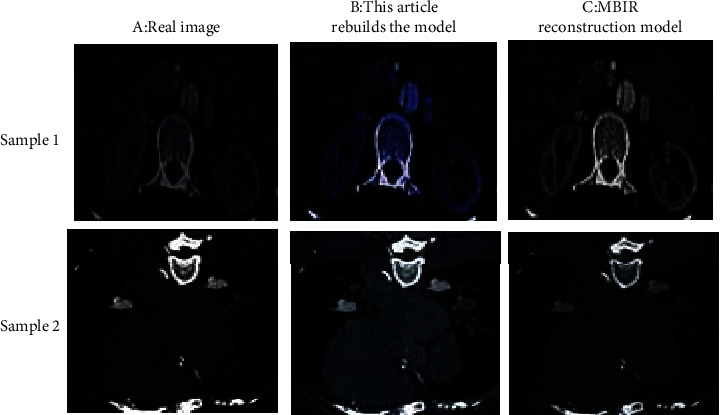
3D reconstruction of the cross-sectional CT image of the heart. (a) Showed the real image; (b) showed the image processed by the reconstruction model proposed; (c) showed the image processed by the MBIR model.
